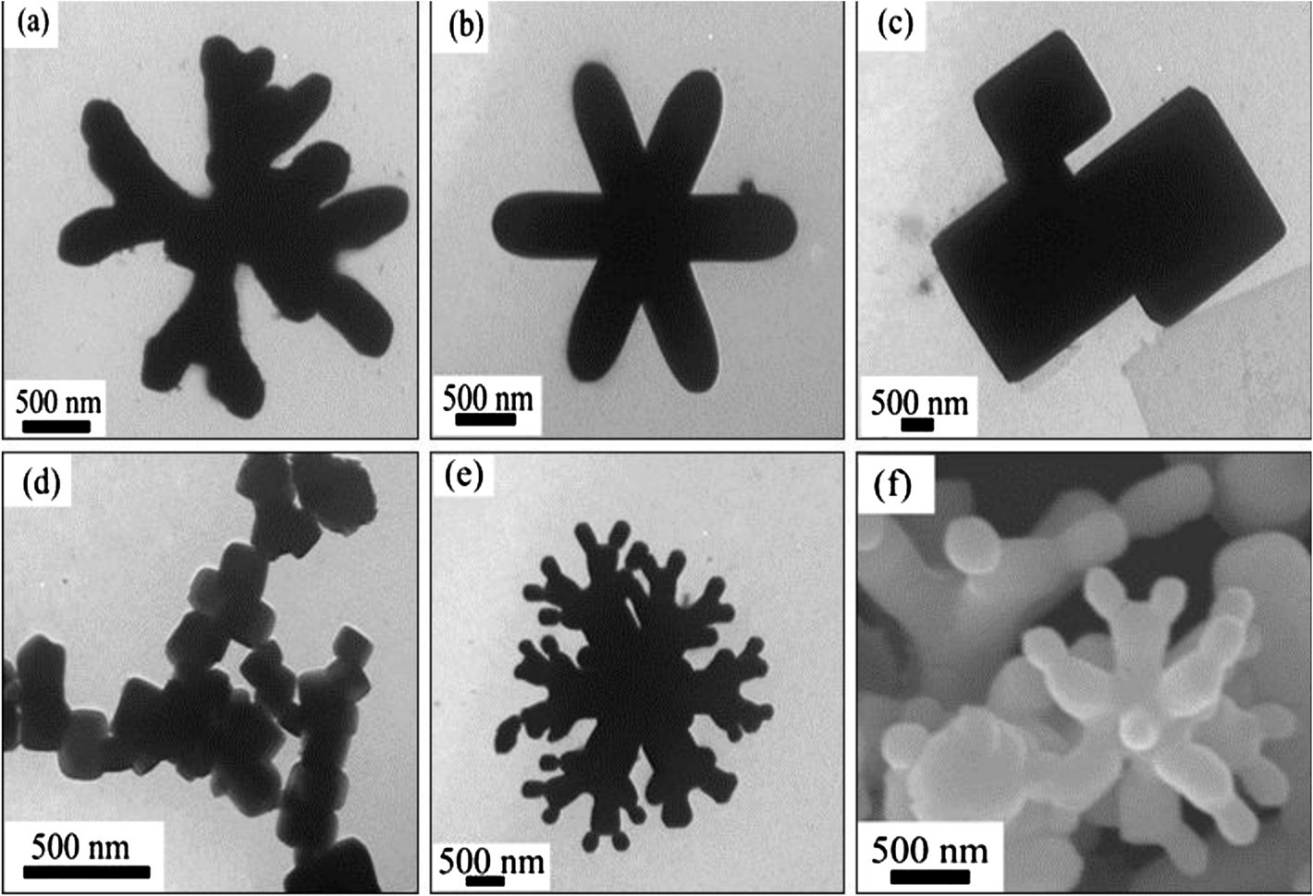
TEM and SEM images of the PbS microstructures. Obtained with different surfactants via solvothermal processes at 180°C for 12 h, respectively. (a) CTAB, (b) SDS, (c) EDTA, (d) CTA, and (e and f) PEG-400.
